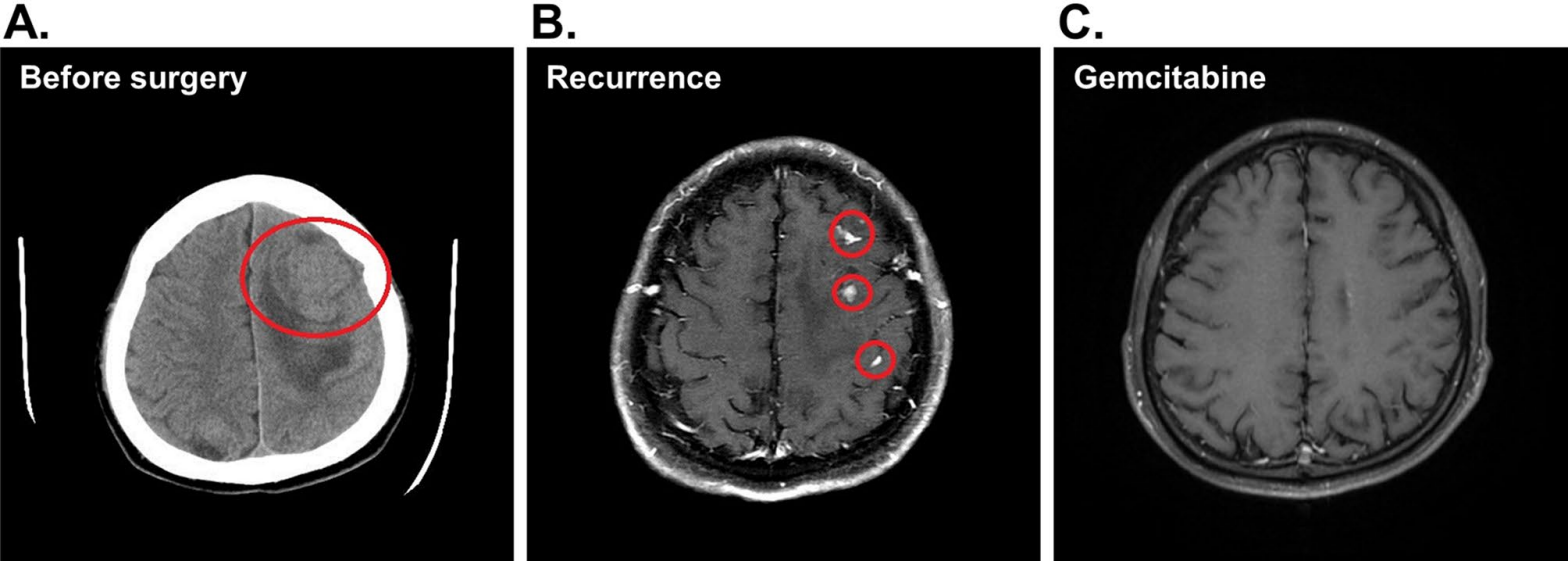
Radiological response of the brain lesion of patient #2 to gemcitabine. (A) A computed tomography (CT) scanning before surgery; the tumor areas are circled. (B) Residual lesion revealed by MRI of patient’s brain in a follow-up after surgery. (C) Follow-up axial MRI of the brain. The patient shows complete response after gemcitabine treatment.
